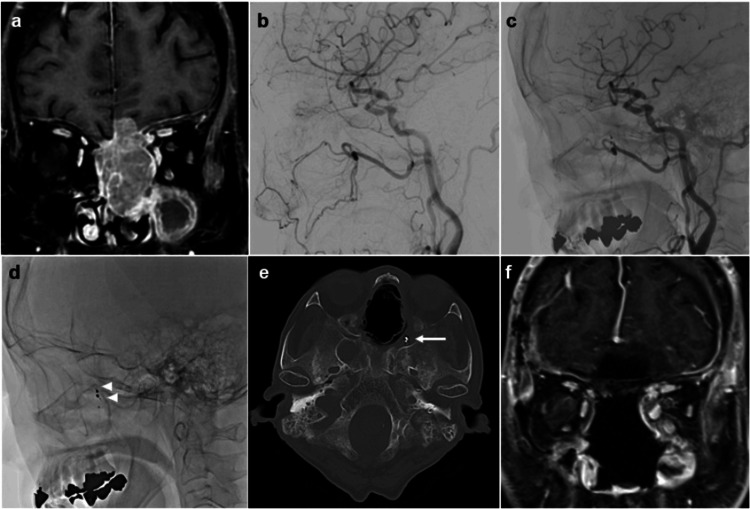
Pre- and postoperative multimodal imaging of Case 2 (malignant sinonasal neuroendocrine carcinoma). (a-f) Preoperative MR image and preoperative angiography demonstrate hypervascularity of the tumor supplied by the sphenopalatine artery (a, b). Post-embolization angiography confirms disappearance of the tumor blush and show complete devascularization (c and arrowhead in d). Postoperative CT bone image show coil placement at the feeding branch (arrow in e).
